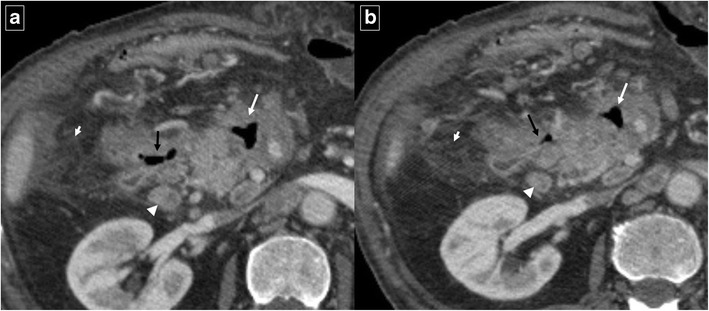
Tuberculosis in a 63-year-old man with constitutional symptoms, fever, and abdominal pain. Axial contrast-enhanced CT shows a masslike appearance (white arrow) that encompasses the superior mesenteric artery and a fistulous pathway that communicates with the duodenal lumen (black arrow). Note the marked extrinsic compression of the third portion of the duodenum. CT also shows periduodenal fat stranding (short arrows) and a necrotic lymph node (arrowhead)
